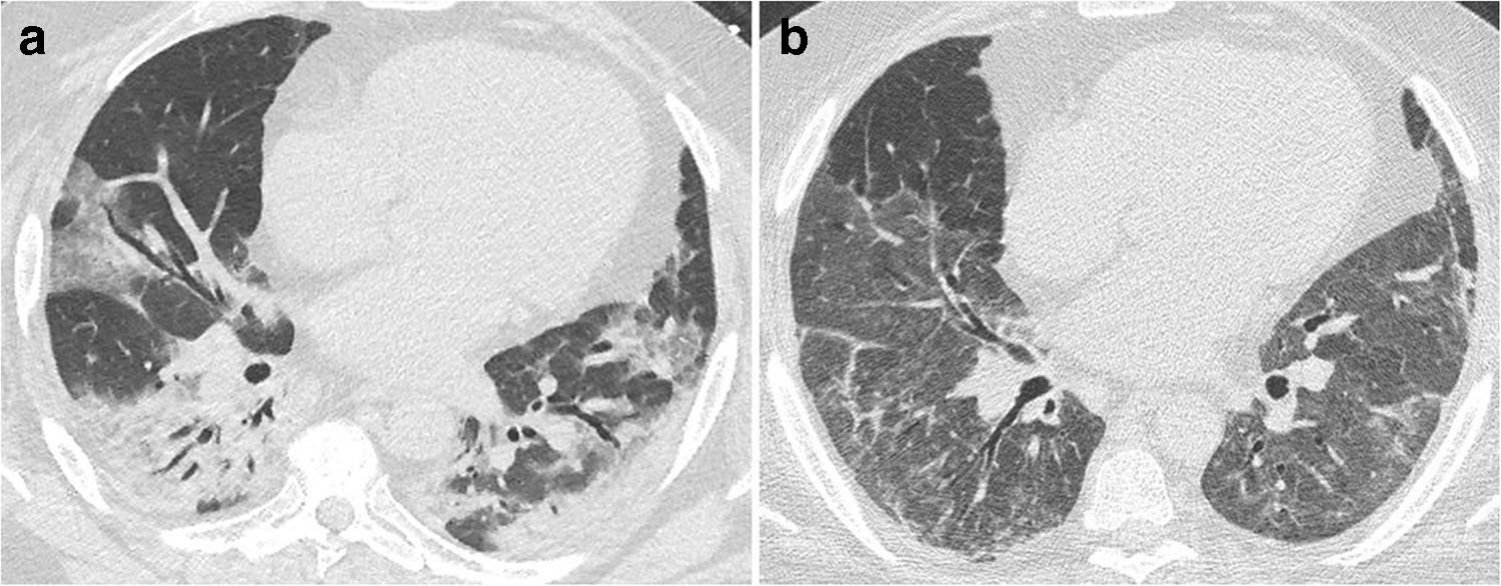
CT features at baseline and at discharge in a 52-year-old man who developed severe COVID-19 pneumonia (intubation, extracorporeal membrane oxygenation, 45 days in ICU). a Baseline CT showing bilateral consolidation and ground glass opacities (GGOs) with peripheral and peribronchovascular distribution. b 2-month CT follow-up demonstrating the complete resolution of consolidation, with residual parenchymal bands bilaterally, while GGOs appear more extensive (“tinted sign”) in the lower lobes, lingula, and right middle lobe. Note the improved expansion of the left lung in b
